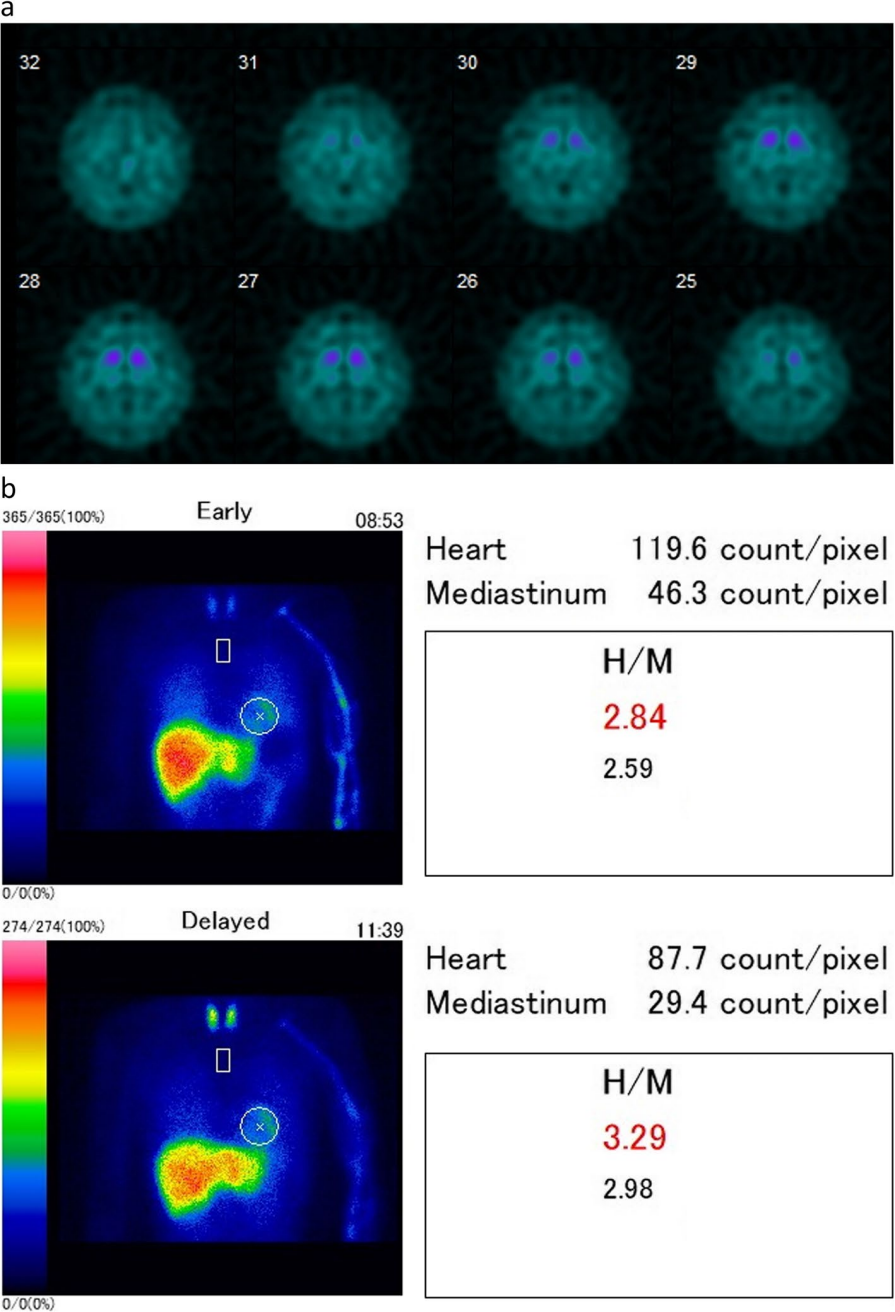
a Dopamine transporter (DAT) scan (dopamine transporter single-photon emission computed tomography [SPECT] using ^123^I-Ioflupane) shows a clear hypo-accumulation in the bilateral striatum with specific binding ratios (SBR) of 2.46 and 2.85 on the right and left sides, respectively. The asymmetric index was 14.8%. b
